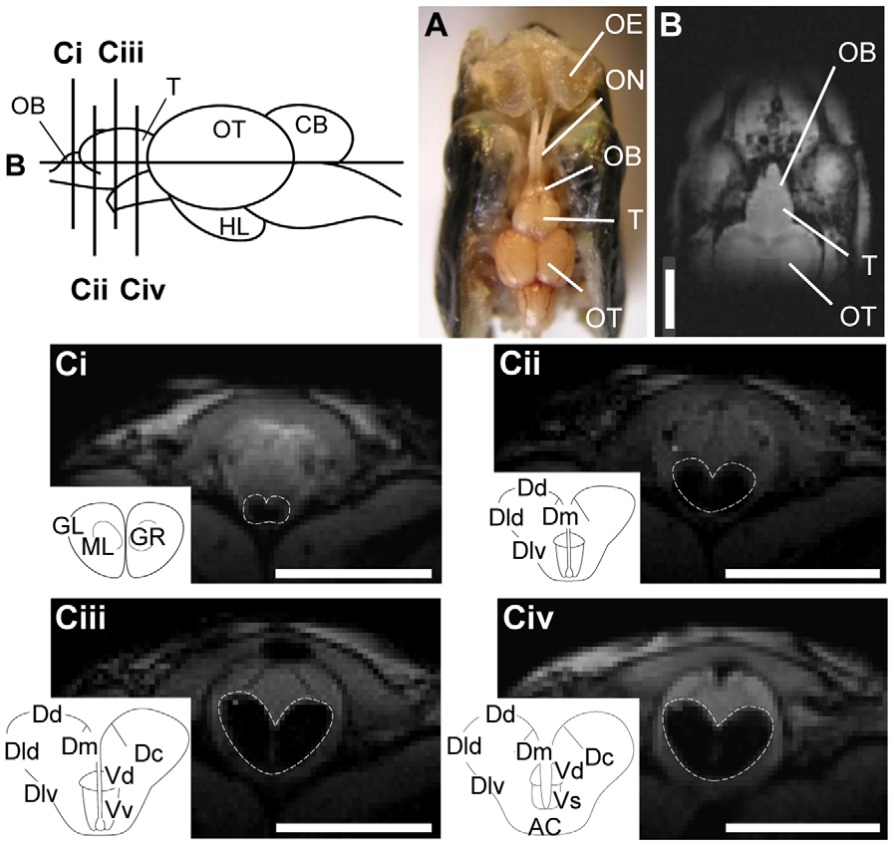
Magnetic resonance imaging (MRI) scan and dissected view of the front part of a lacustrine sockeye salmon head. *A*: Dissected view; *B*: MRI horizontal section; *Ci–iv*: serial MRI scan of frontal sections of the olfactory bulb (*Ci*) and the telencephalon (*Cii–iv*). The olfactory bulb and telencephalon are outlined by dotted lines. Scale bars = 5.0 mm. AC, anterior commissure; CB, cerebellum; Dc, central area of dorsal telencephalon; Dd, dorsal area of dorsal telencephalon; Dld, dorsal part of lateral area of dorsal telencephalon; Dlv, ventral part of lateral area of dorsal telencephalon; Dm, medial area of dorsal telencephalon; GL, glomerular layer; GR, granular layer; HL, hypothalamus; ML, mitral cell layer; OB, olfactory bulb; OE, olfactory epithelium; ON, olfactory nerve; OT, optic tectum; T, telencephalon; Vd, dorsal area of ventral telencephalon; Vs supracommissural area of ventral telencephalon; Vv, ventral area of ventral telencephalon.
